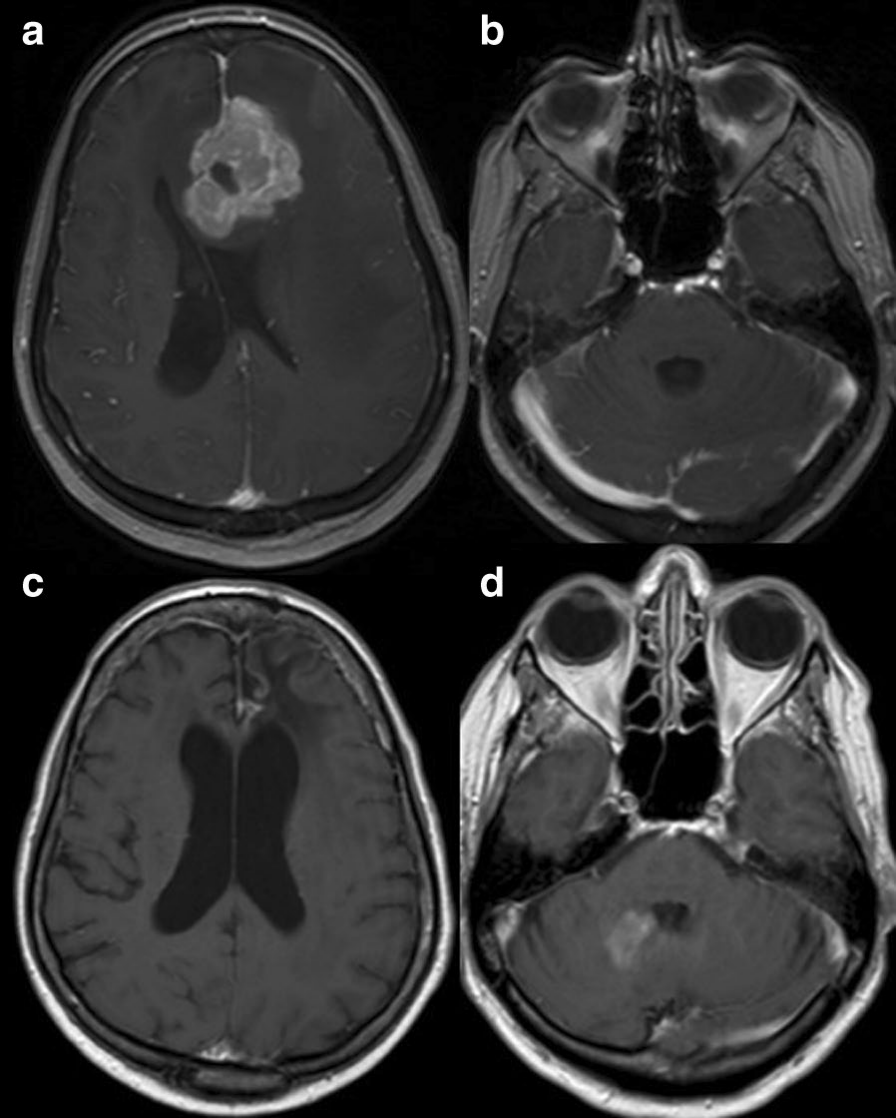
Case 3; Axial T1-weighted post contrast imaging at initial presentation (a, b), after completing therapy with HD-MTX (c) and relapse (d). Initial presentation shows a large enhancing lesion in the left frontal lobe (a) and normal cerebellum (b). Patient was in complete response after completing 1 year of HD-MTX treatment (c) with radiographic relapse in the right cerebellum, 6 years after the initial diagnosis (d)
